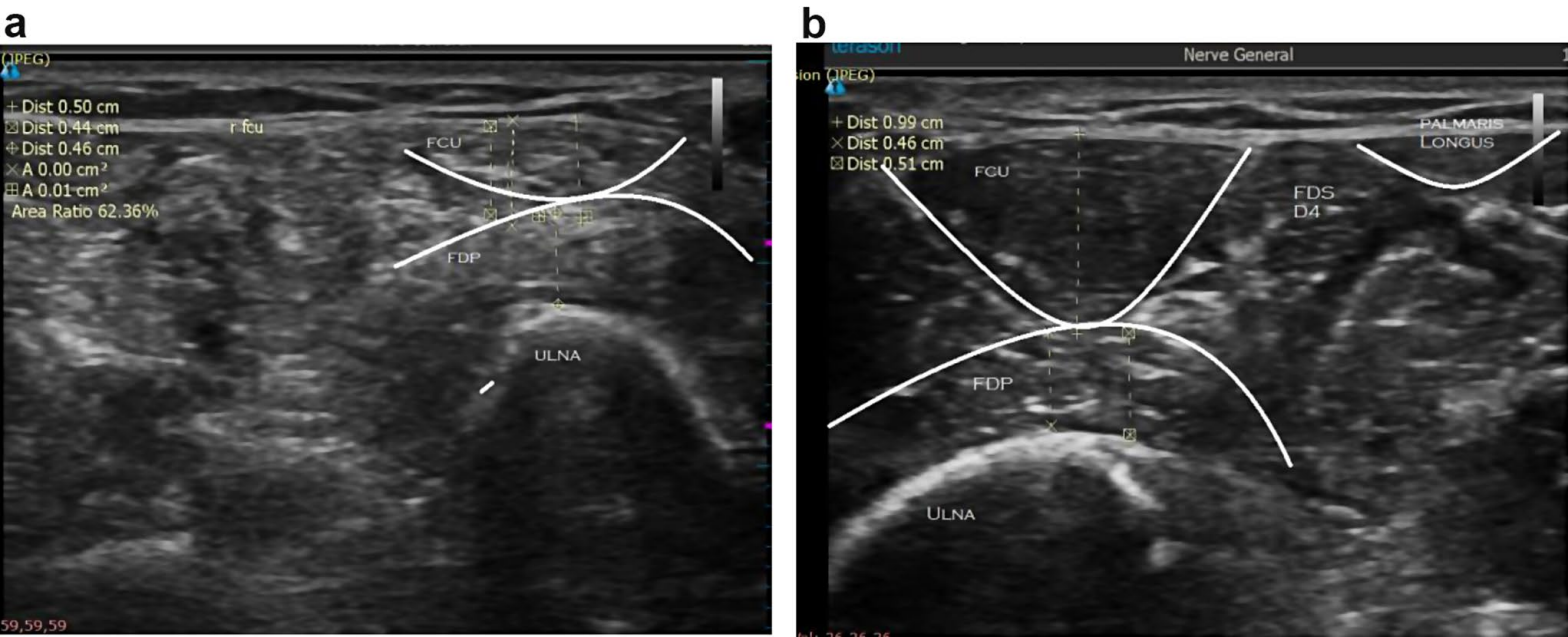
(a) Ultrasound shows atrophy of the flexor carpi ulnaris muscle (width 0.50 cm) in the affected side 3 months after injection. (b) Ultrasound of normal flexor carpi ulnaris muscle (width 0.99 cm) in the unaffected side 3 months after injection
